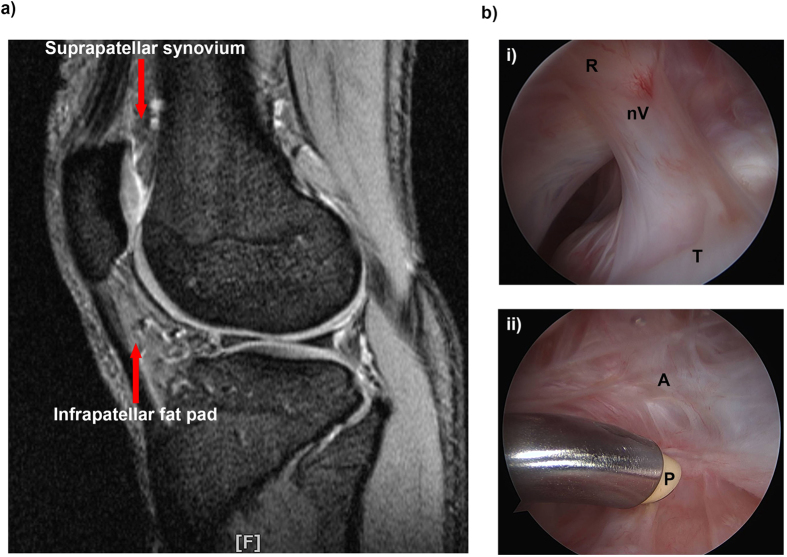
Arthroscopic evidence of arthrofibrosis in the knee. (a) Magnetic resonance image (MRI) of a knee joint. Red arrows indicate the location of the suprapatellar synovium and infrapatellar fat pad. (b) Arthroscopic view of an aberrant fibrous band bridging the space between the suprapatellar pouch roof (R) with evidence of neovascularisation (nV) and the superior articular margin of the trochlea (T) in a patient with severe arthrofibrosis (i). Surgical cautery with a diathermy probe (P) of dense layered adhesions (A) lying within the lateral synovial gutter of the knee (ii).
